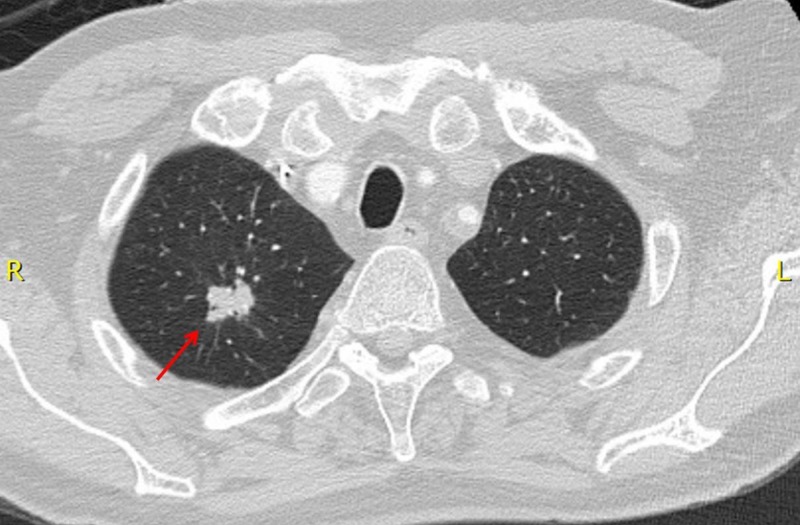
CT chest, pre-SBRT, demonstrating the spiculated 1.8 cm adenocarcinoma (NSCLC) of the right upper lobe of the lung. NSCLC, non-small cell lung cancer.
